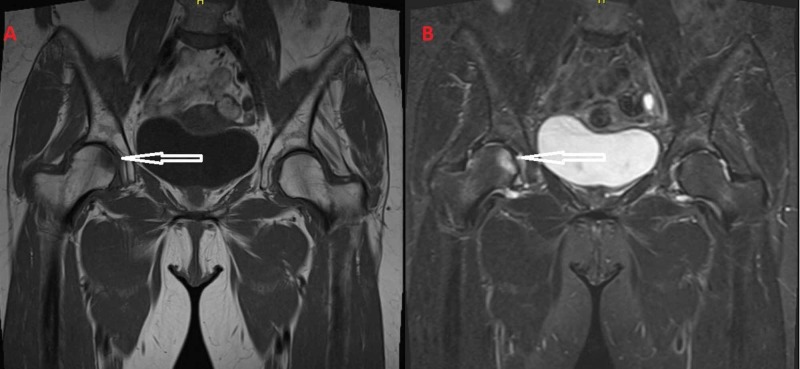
(A) Initial T1-weighted images and (B) initial T2-weighted images of the MRI of the right hip reveal the bone marrow edema of the right femoral head.
